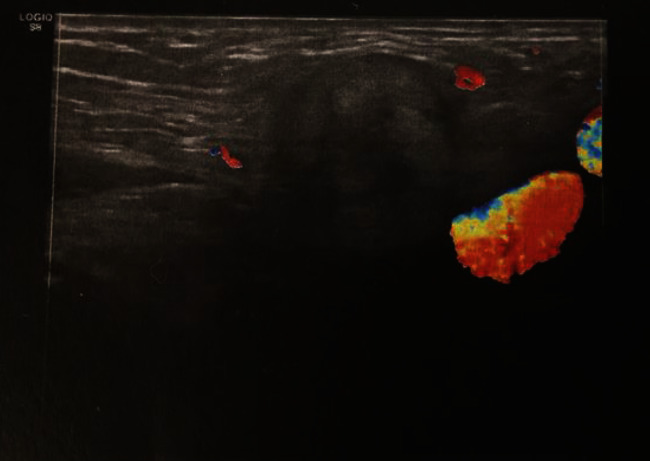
Tripplex ultrasonography: ultrasound findings suggesting left inguinal hernia (arrow).
